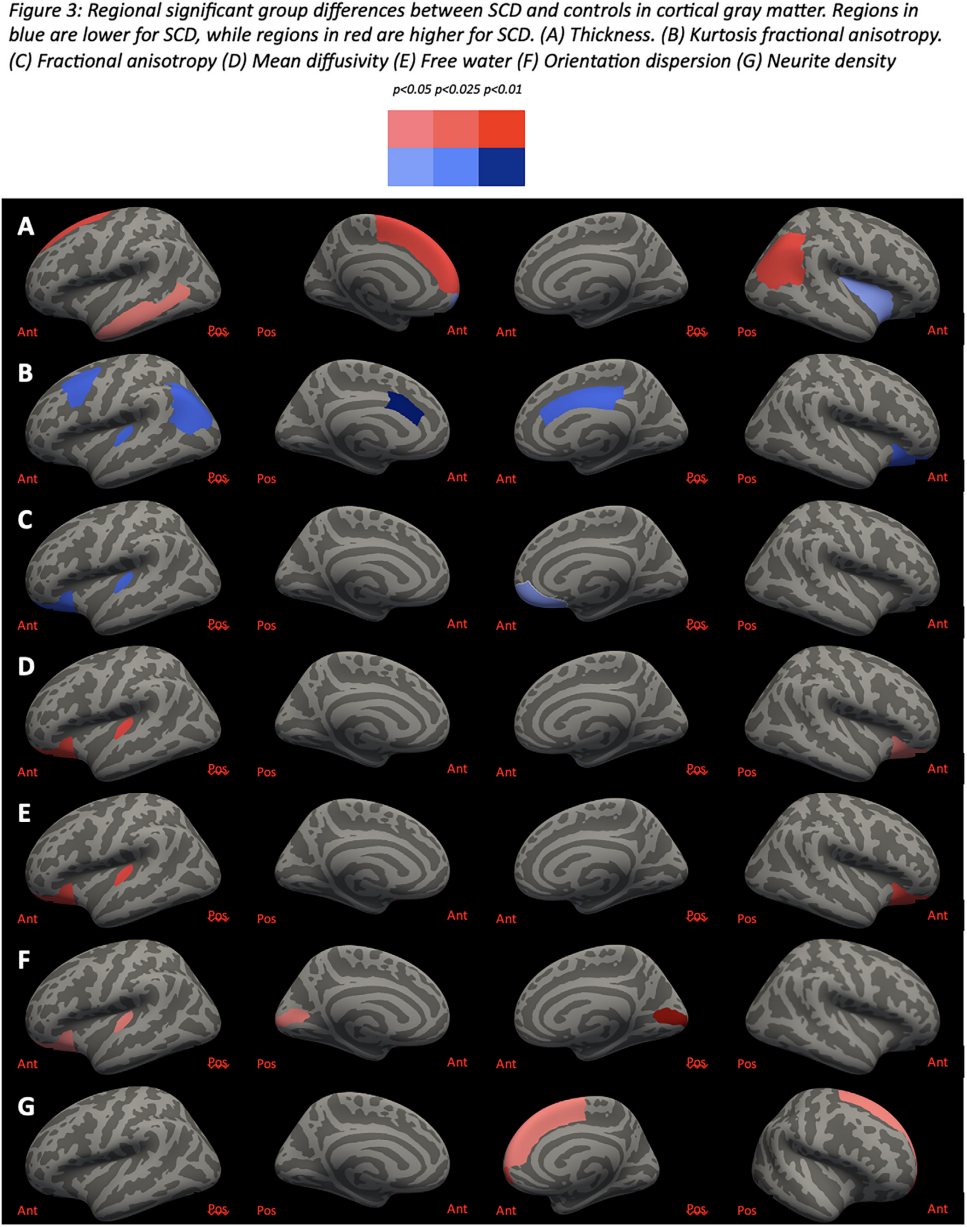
# A diffusion kurtosis MRI signature of subjective cognitive decline
